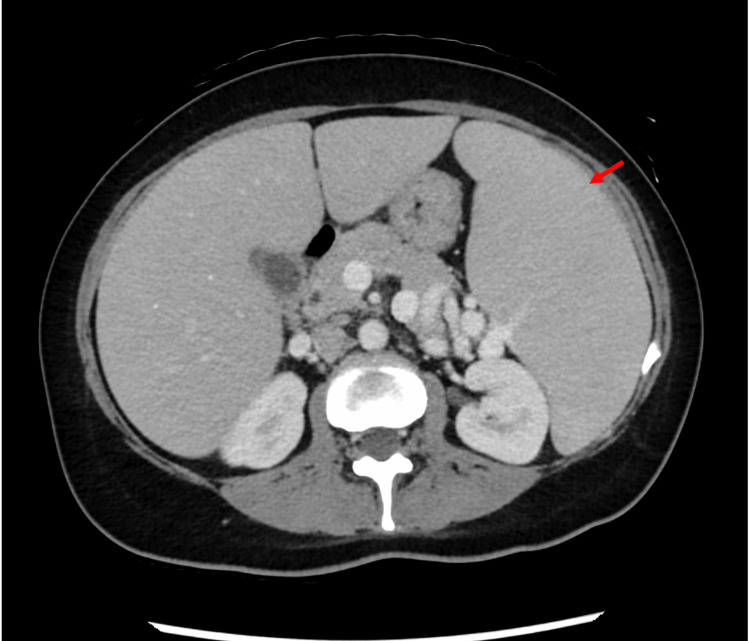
CT abdomen and pelvis CT abdomen and pelvis with contrast showing splenomegaly, as identified by the red arrow.
